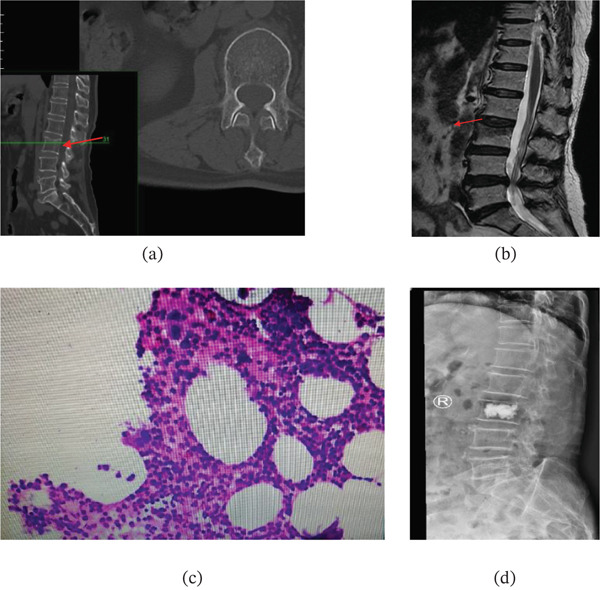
Imaging and pathology of the patient in Case 1 (pyogenic spondylitis misdiagnosed as OVF). (a) Preoperative sagittal CT showed no obvious bone destruction at L3. (b) Sagittal T2‐weighted/STIR MRI showed L3 vertebral marrow edema and compression fracture without discitis, epidural extension, or paravertebral soft‐tissue abnormality—findings that contributed to the initial misdiagnosis of a benign OVF. (c) Intraoperative vertebral bone marrow aspiration biopsy during PVP showed suppurative inflammation, confirming pyogenic spondylitis. (d) Postoperative lateral radiograph showed satisfactory cement filling after L3 PVP.
